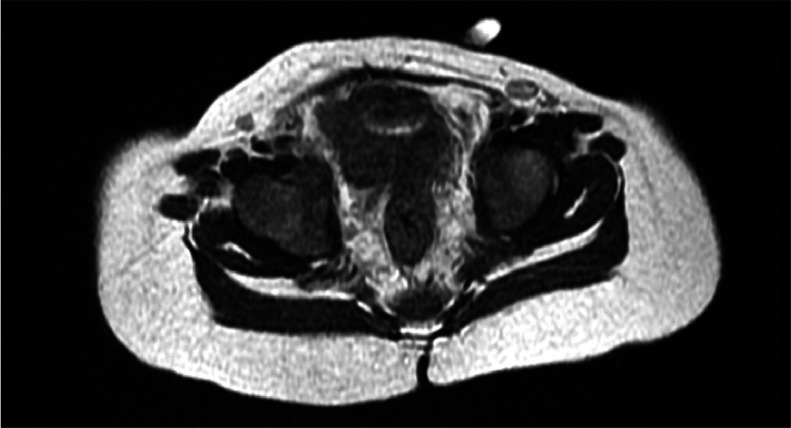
The axial plane T2-weighted MR image shows the complete formation of the sphincter ani in the middle.
